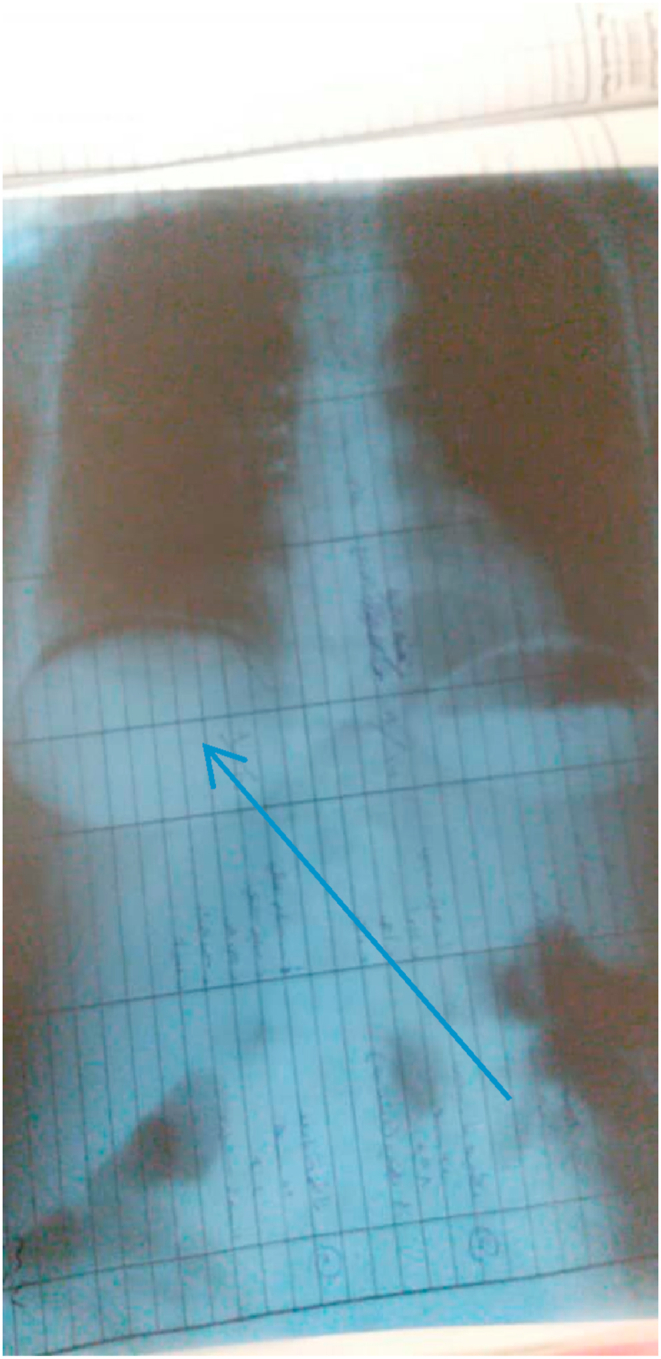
Preoperative X-ray images. Free air under the diaphragm which referred to perforation.
